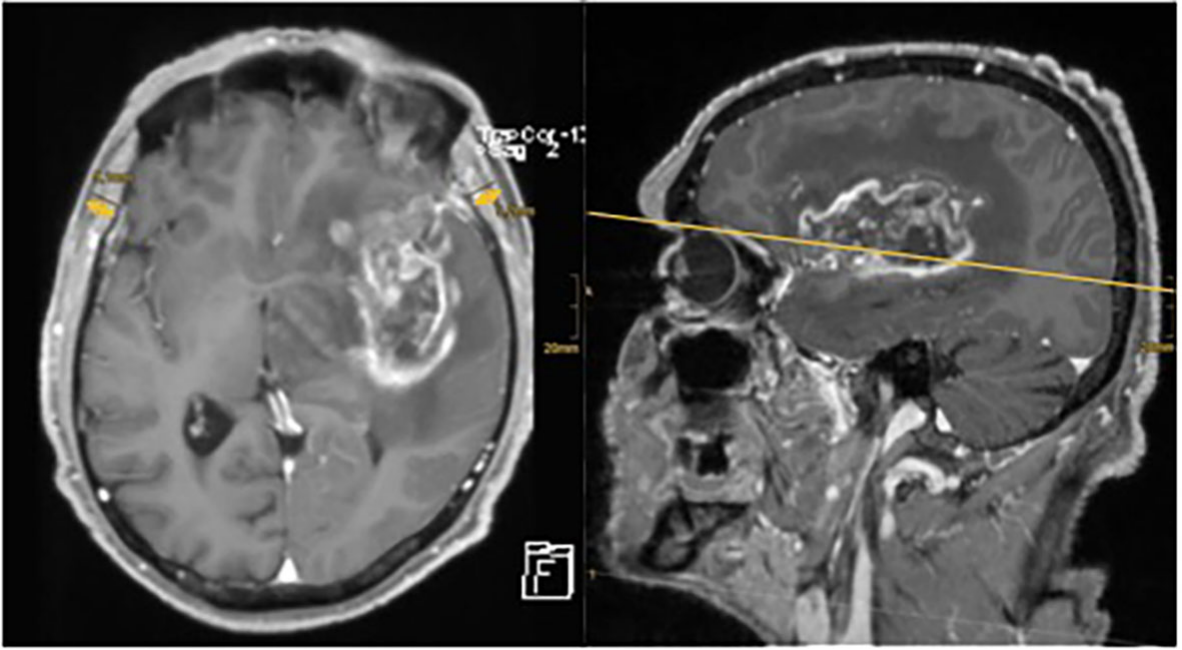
Axial and sagittal MRI images of one of the neuro-oncological database’s patient. There is a large contrast enhancing tumor visible in the left frontotemporal hemisphere. TMT has been measured on the axial plane using the Sylvian fissure and orbital roof as reference points. The arrows indicate the extent of TMT measurement.
